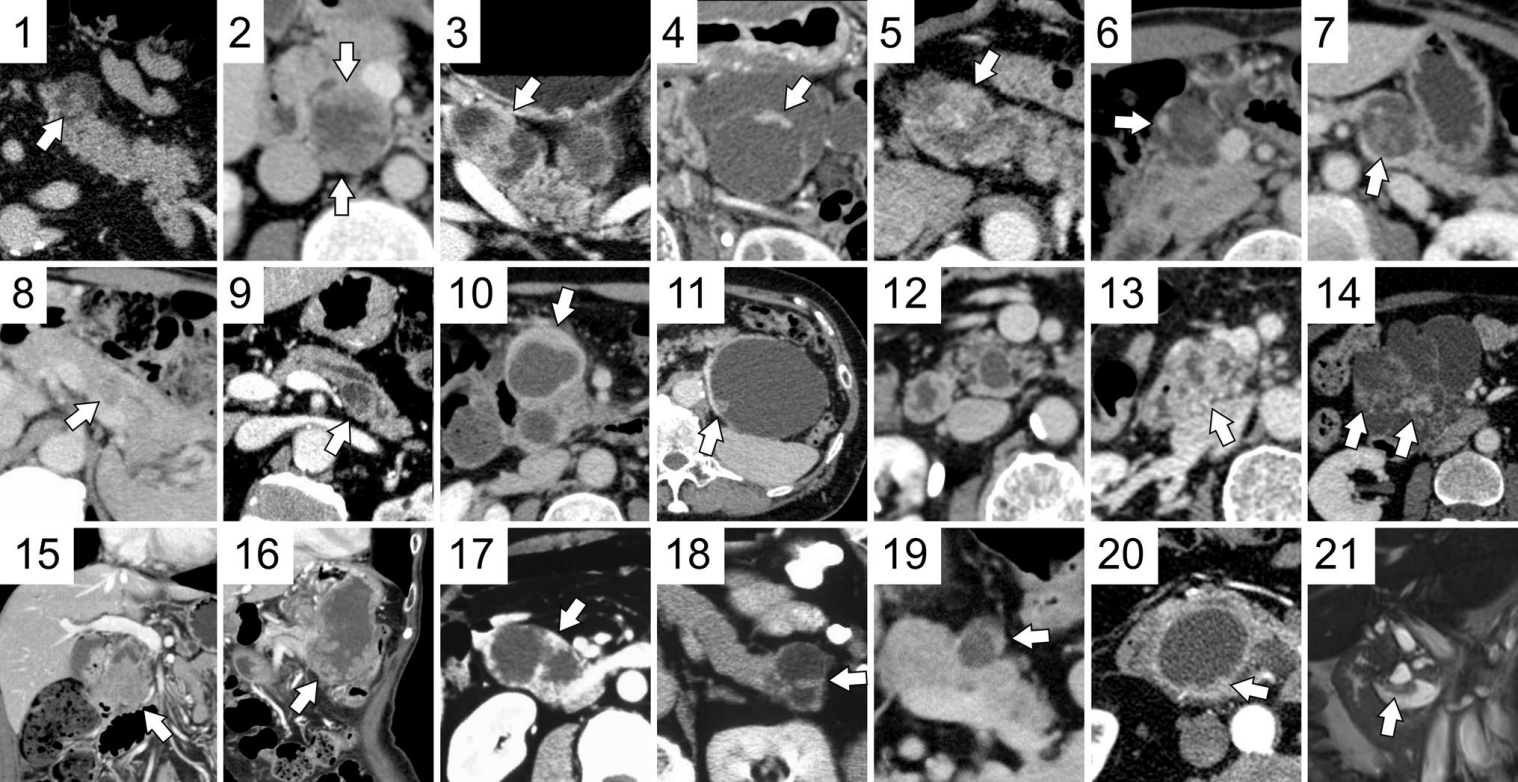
Cases 1–21 of intraductal oncocytic papillary neoplasm of the pancreas are listed with contrast-enhanced computed tomography images. Arrows show solid parts. Coronal views show the location of duodenal involvement in Case 15 and gastric and transverse colon involvement in Case 16. In Case 21, a coronal image of steady-state free precession has been presented because contrast-enhanced computed tomography was not obtained.
